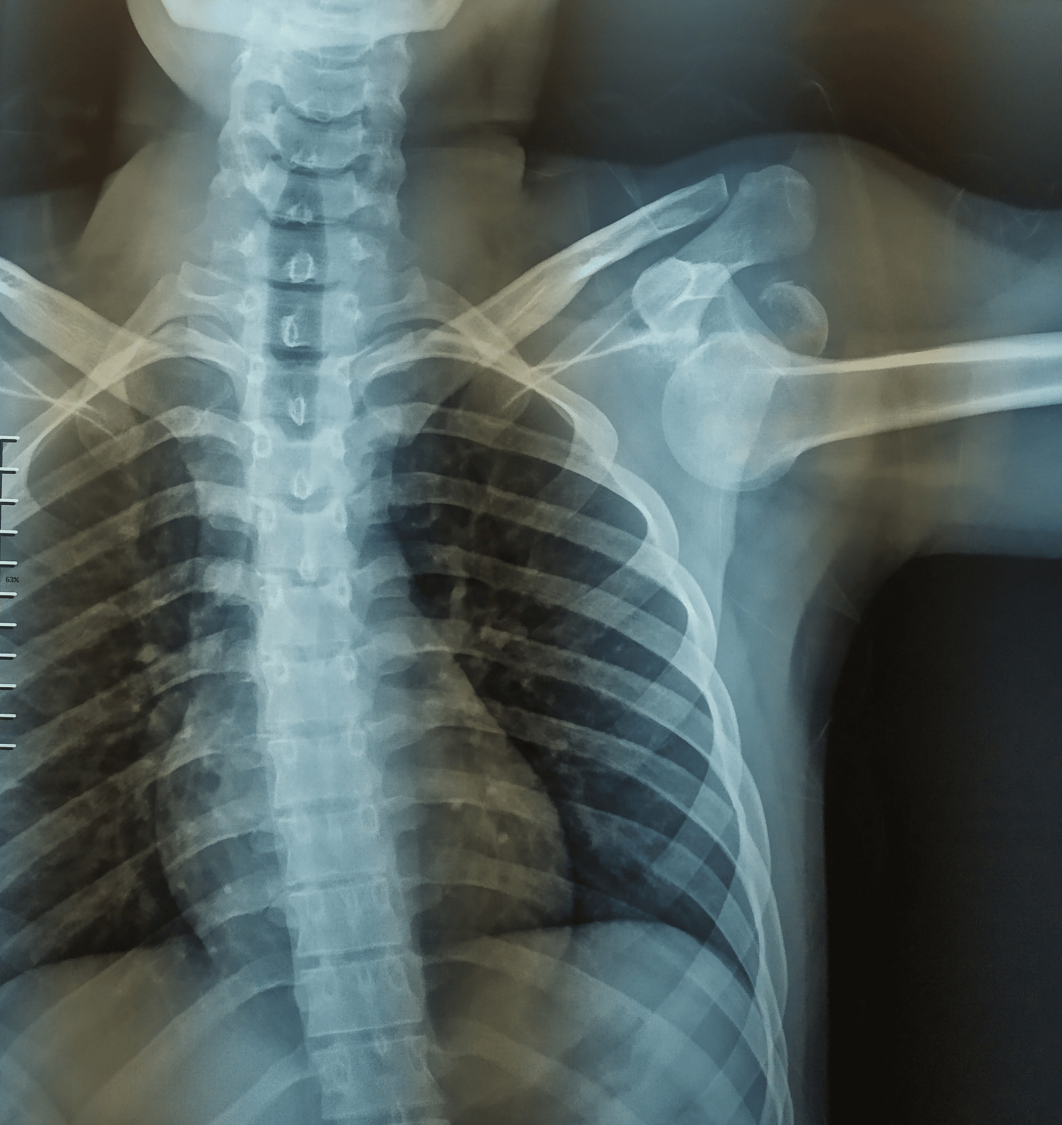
Anteroposterior X-ray of the shoulder showing luxatio erecta with the humeral head displaced inferiorly to the glenoid.
